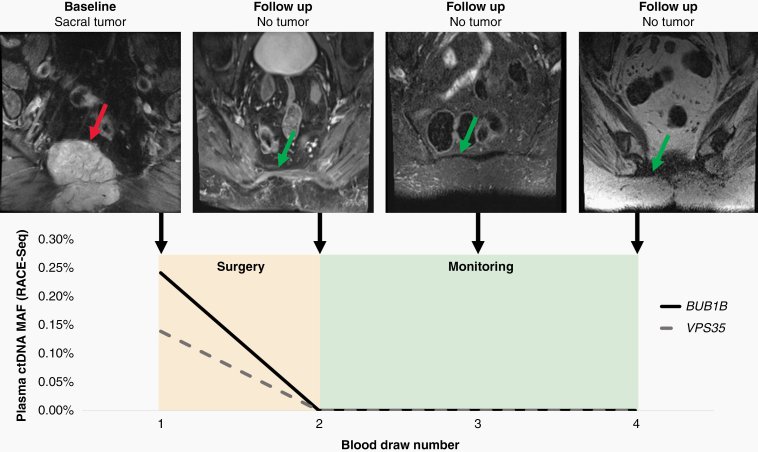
Liquid biopsy using RACE-Seq to detect mutations in *BUB1B* and *VPS35* demonstrates concordance with radiographic monitoring during the follow-up period for patient 8564. At the time of diagnosis, RACE-Seq detected mutations in *BUB1B* and *VPS35* that were concordant with whole exome sequencing data from the primary sacral tumor. After surgical resection, patient 8564 returned for three additional follow-up visits where no mutations were detected in plasma, which correlates with no detectable recurrence by MRI. The red arrow identifies the primary sacral tumor, while the green arrows demonstrates no tumor recurrence at the primary site.
